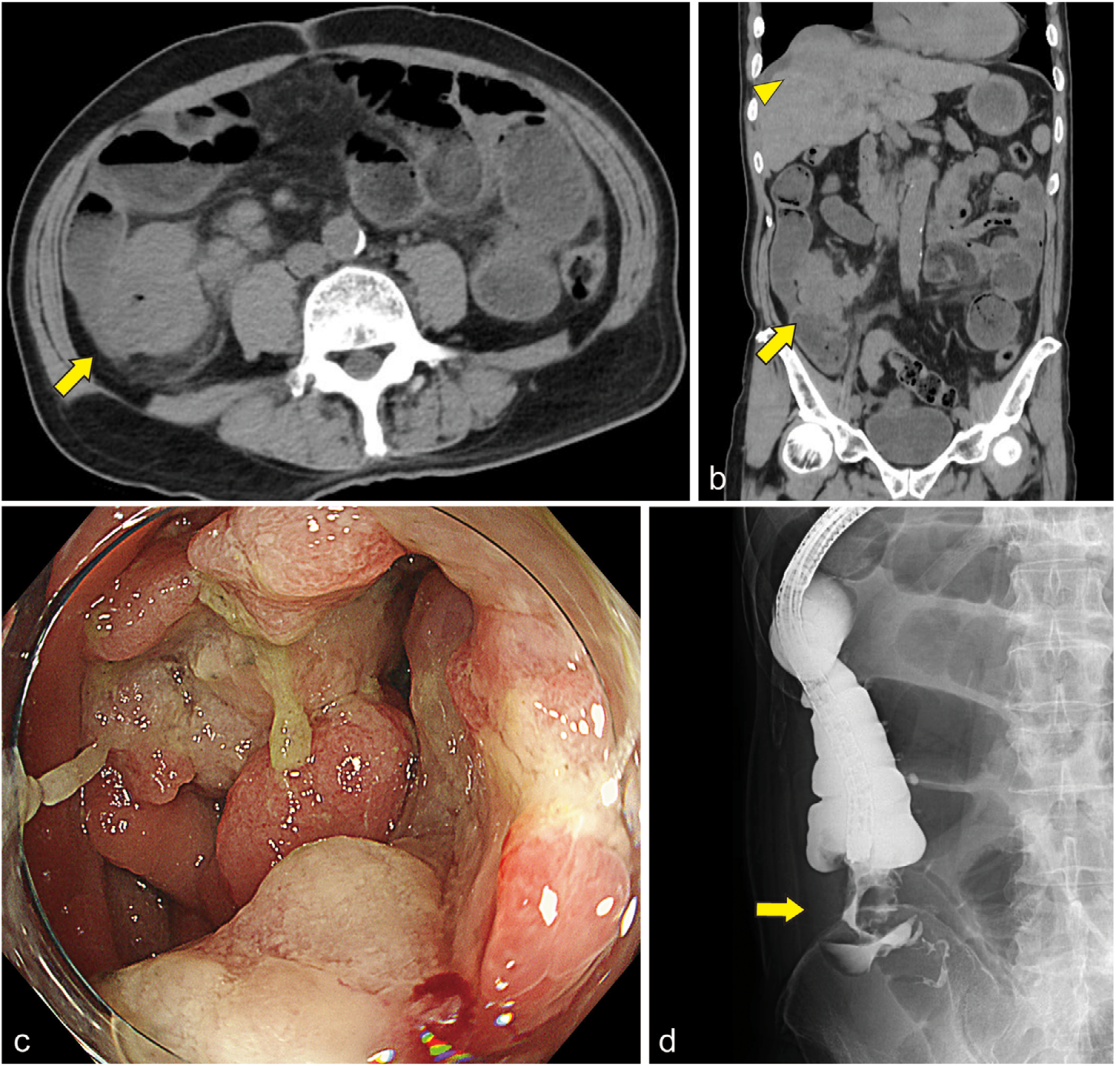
Computed tomography scan showing thickened walls in the ileocecal region with proximal bowel dilatation (arrows). (a) Axial view. (b) Coronal view. The liver shows signs of cirrhosis and metastatic tumors (arrowhead). (c) Endoscopy showing a tumor with severe stenosis in the ileocecal region. (d) Fluoroscopy showing stenosis of the ileocecum, wherein the contrast medium does not flow into the ileum (arrow).
